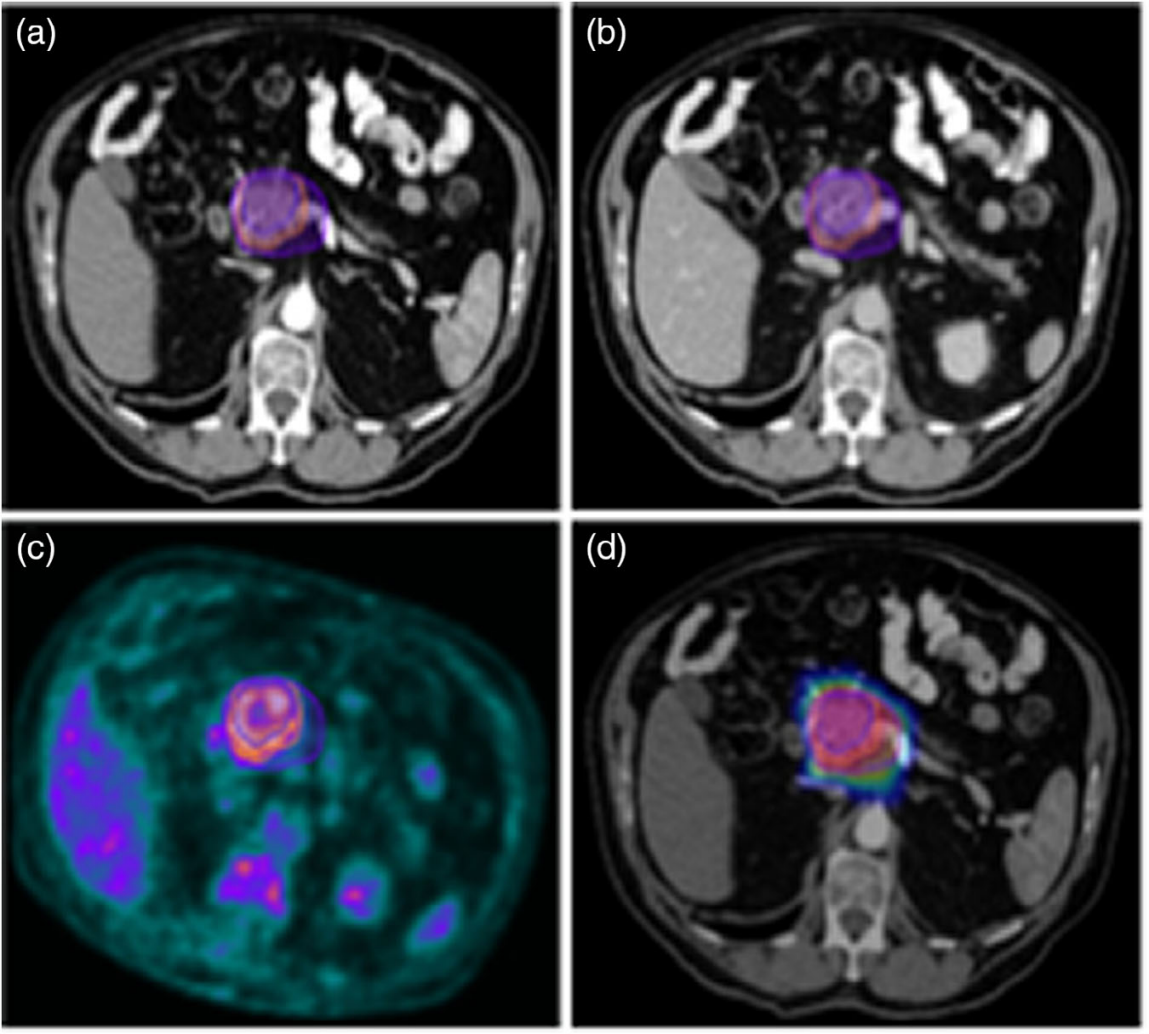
A 76‐year‐old male with borderline resectable adenocarcinoma of the pancreatic head. Images procured from our institution. (a) Axial view of the planning CT in arterial phase showing gross tumor volume (GTV) and planning target volumes (PTV 4000 and PTV 3300) contours. (b) Axial view of the planning CT in venous phase with contrast highlighting GTV, PTV 4000, and PTV 3300. (c) axial view of the PET scan with GTV, PTV 4000, and PTV 3300 contours, window level 0 to 6 SUV. (d) Axial view of the planning CT in arterial phase with GTV, PTV 4000, and PTV 3300 contours, plus radiation therapy (RT) plan dose color wash, dose window level 20 to 40 Gy.
